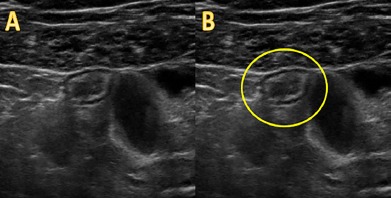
Frame A* is a short-axis view of a point-of-care ultrasound showing an edematous, non-compressible tubular structure in the right lower quadrant of the abdomen with adjacent normal-appearing, compressible loops of small bowel. Clearly visible is the “gut signature” associated with the different layers of the bowel wall; serosa (echogenic), muscularis externa (hypoechoic), submucosa (echogenic), muscularis mucosa (hypoechoic), and mucosa (echogenic). This structure is circled in *Frame B*.
